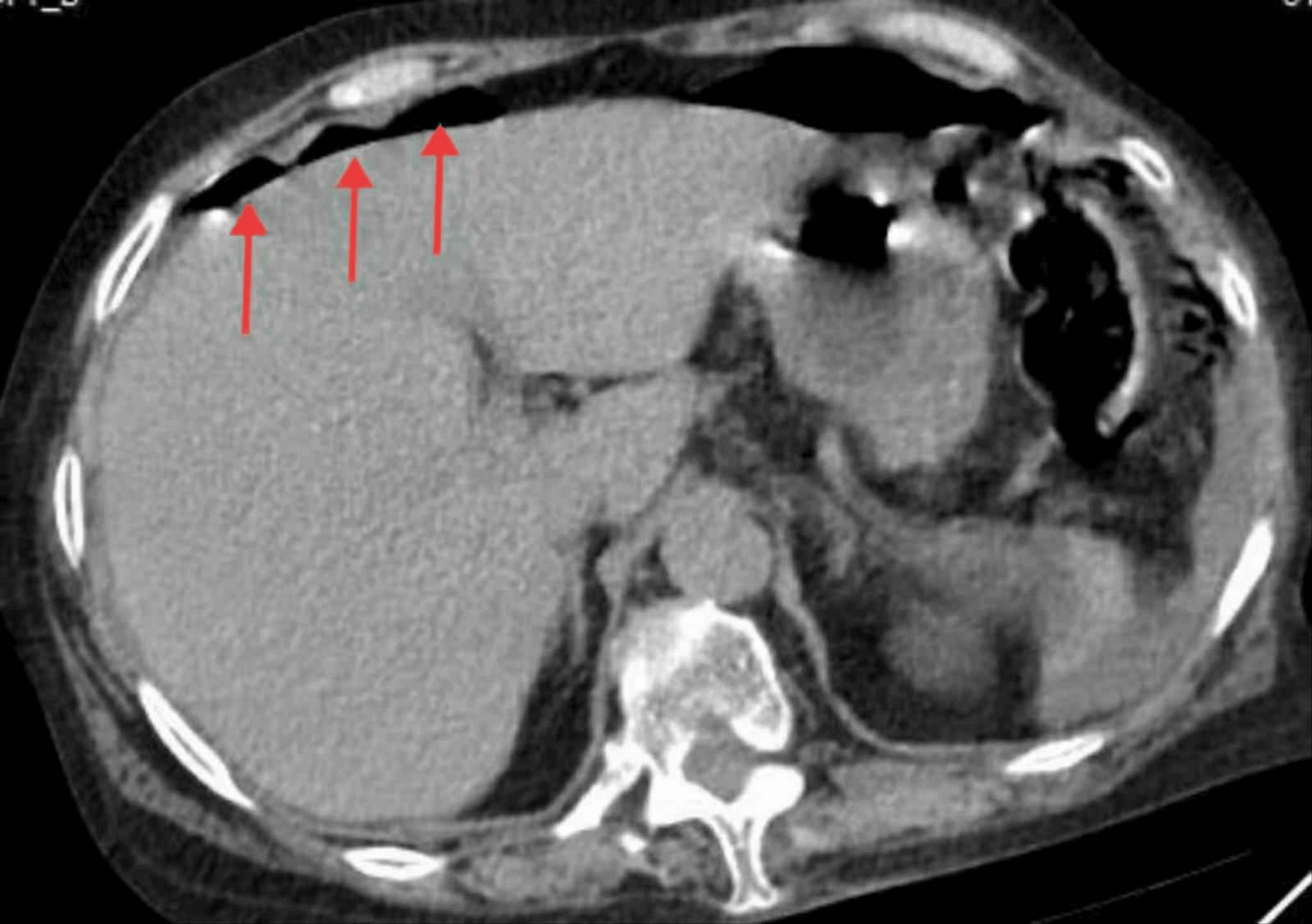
CT scan of the upper abdomen shows a large amount of free gas in the abdominal cavity
